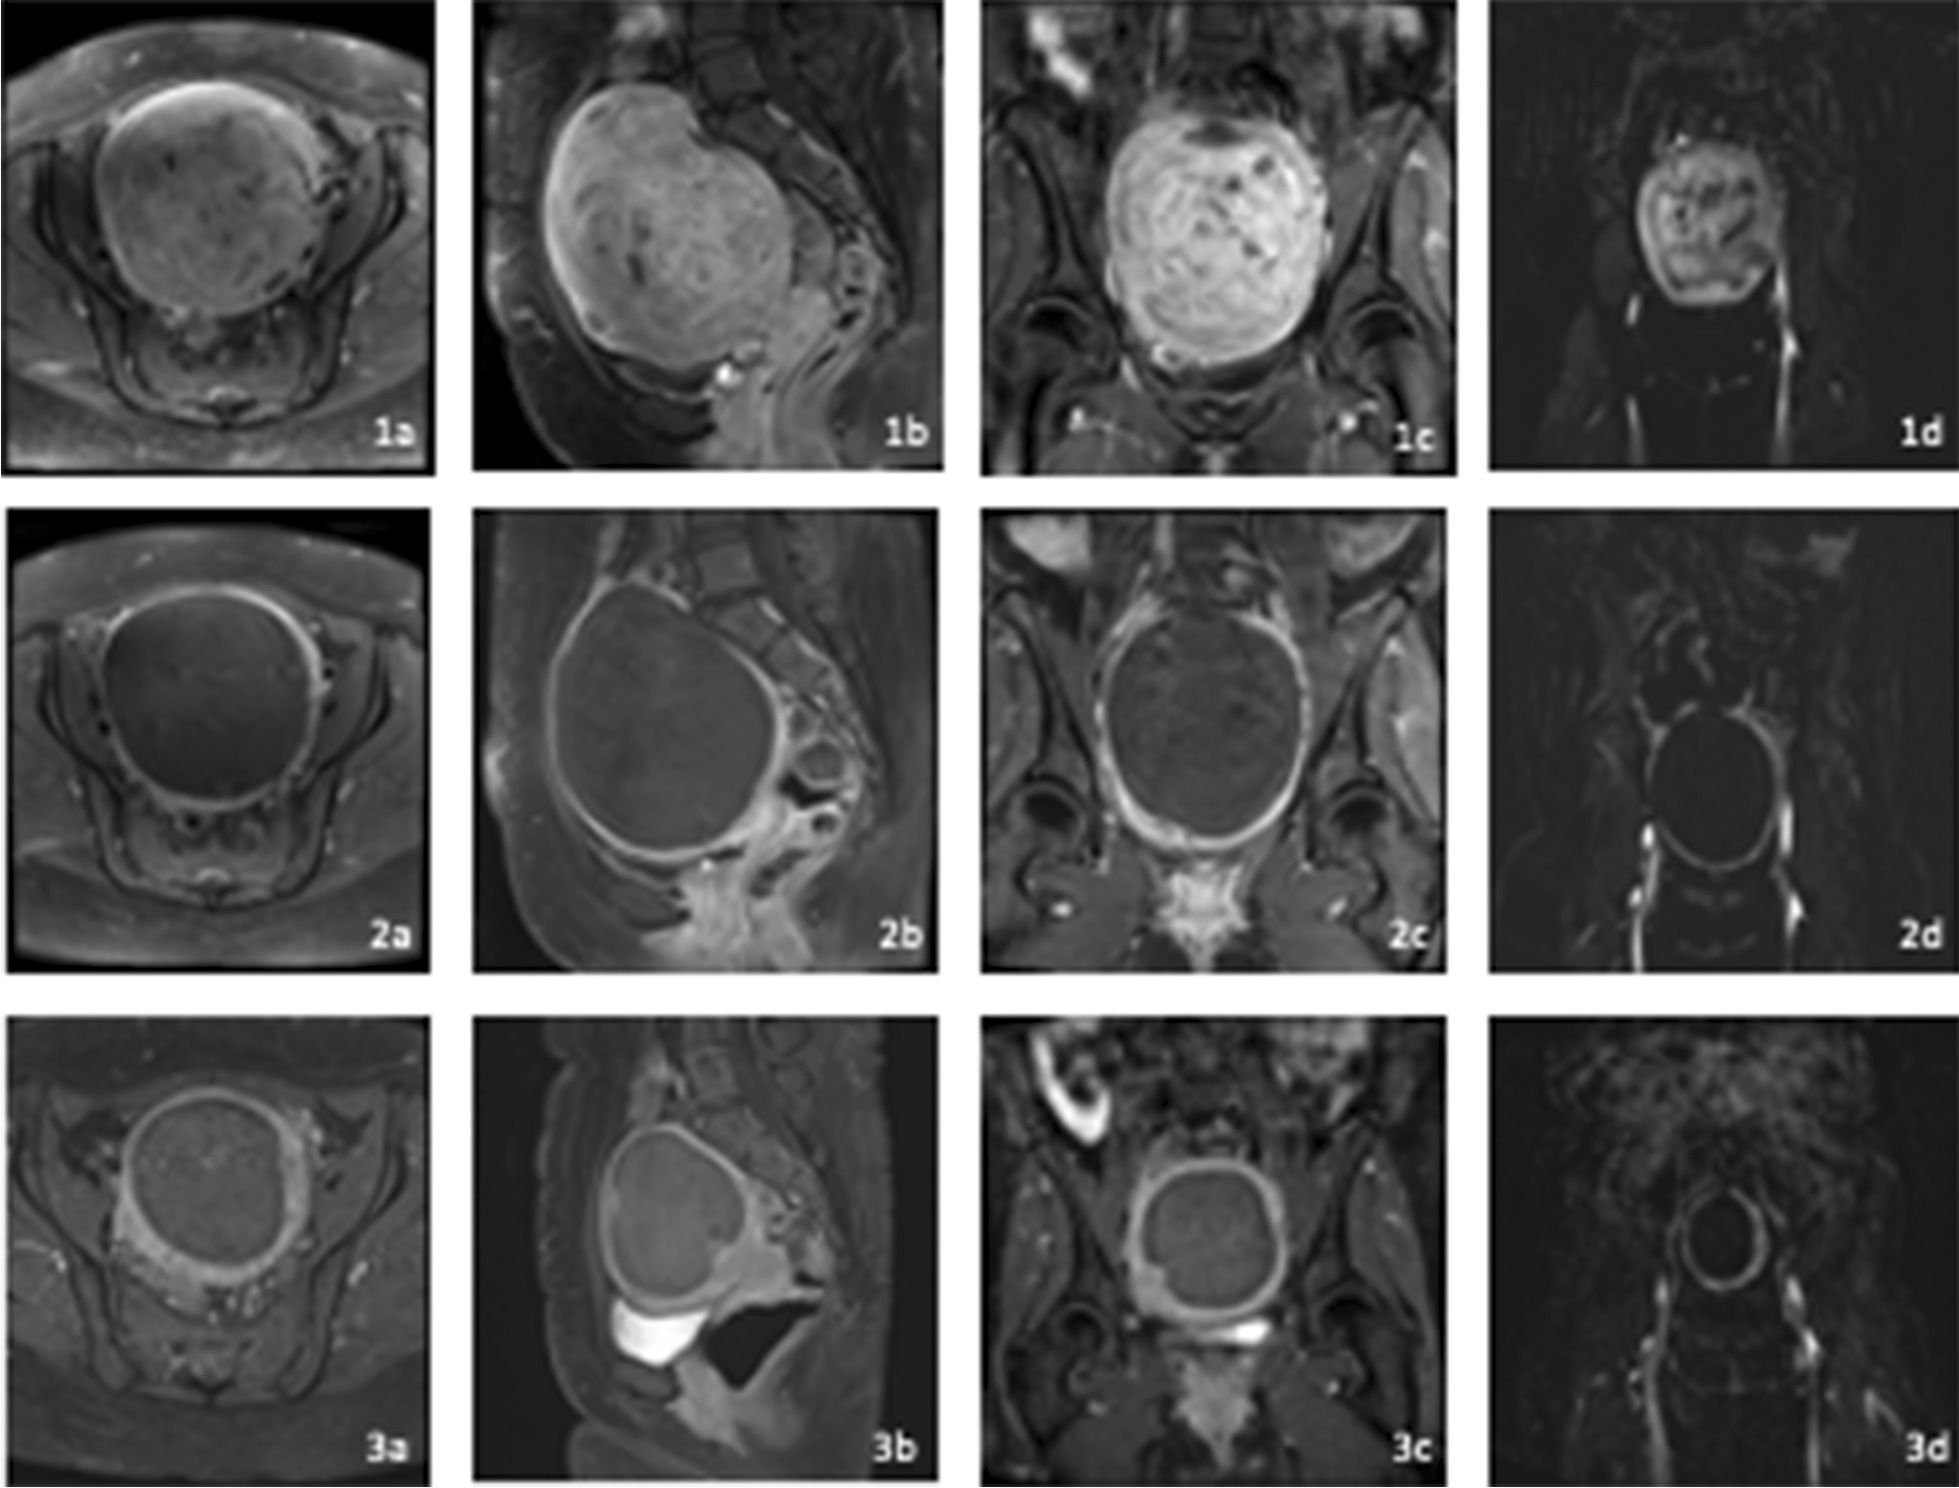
Contrast-enhanced MRI of an extensive uterine fibroid in a symptomatic patient pre (1), post (2) and at 12 month follow-up after UFE (3) a T1 axial, b T1 sagittal, c T1 coronal, d perfusion map in coronal view (blood flow)
